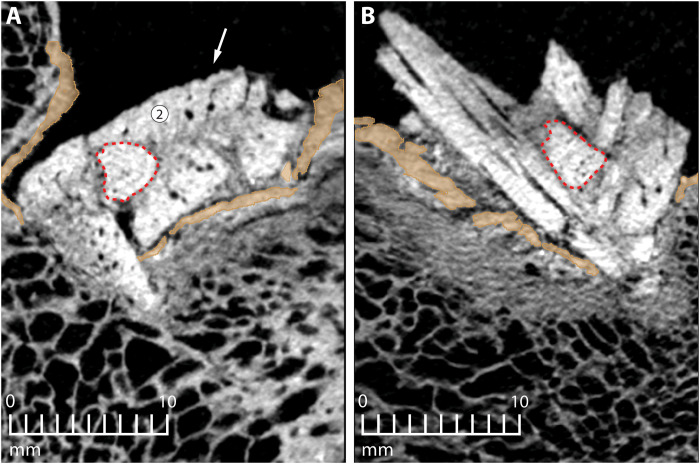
Trabecular bone compaction and bone co-ossification. Displaced cortical bone shown in brown. Red dotted lines outline the cast left behind at the sample location for bone powder used in ancient DNA and protein sequence analysis. (A) CT cross section of embedded fragments (white, high-attenuation cortical bone) demonstrating signs of co-ossification (fine, gray texture, indicating lower attenuation, with some void spaces remaining) between discrete fragments. Arrow points to evidence of external striations on fragment 2. Haversian canals appear as small (<1 mm in diameter), roughly circular, black (air-filled) openings in cortical bone. (B) CT cross section shows signs of healing between discrete fragments from another angle, along with crushed, co-ossified trabecular bone (fine, gray texture, with only rare voids) beneath the displaced cortical bone.
